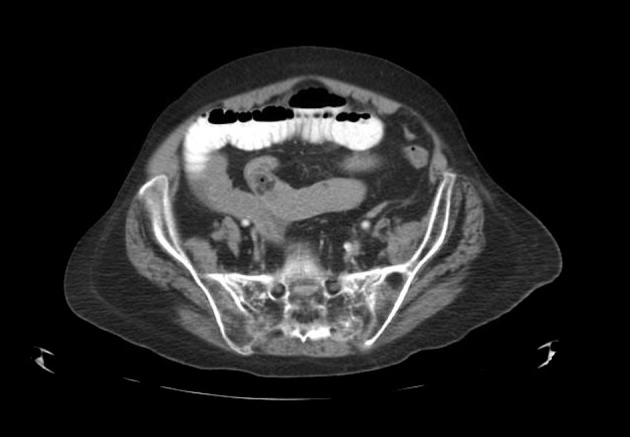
CT scan demonstrating moderate degree of diffuse small bowel dilation with air-fluid levels and in the distal small bowel a suspected intraluminal fatty mass visualized in the small bowel loop in the midpelvis.
